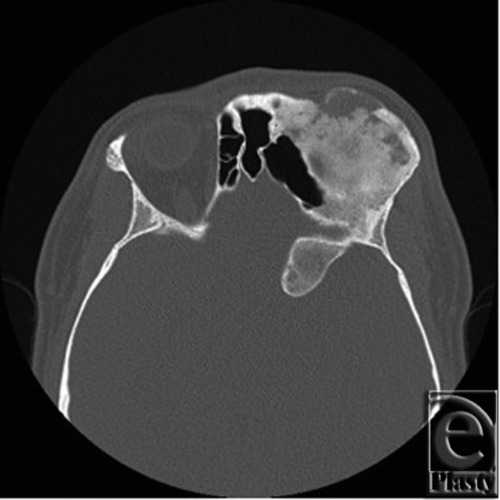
Preoperative axial computed tomographic image exhibiting characteristic ground-glass opacification of fibrous dysplasia involving the left frontal, temporal, and sphenoid bones.
